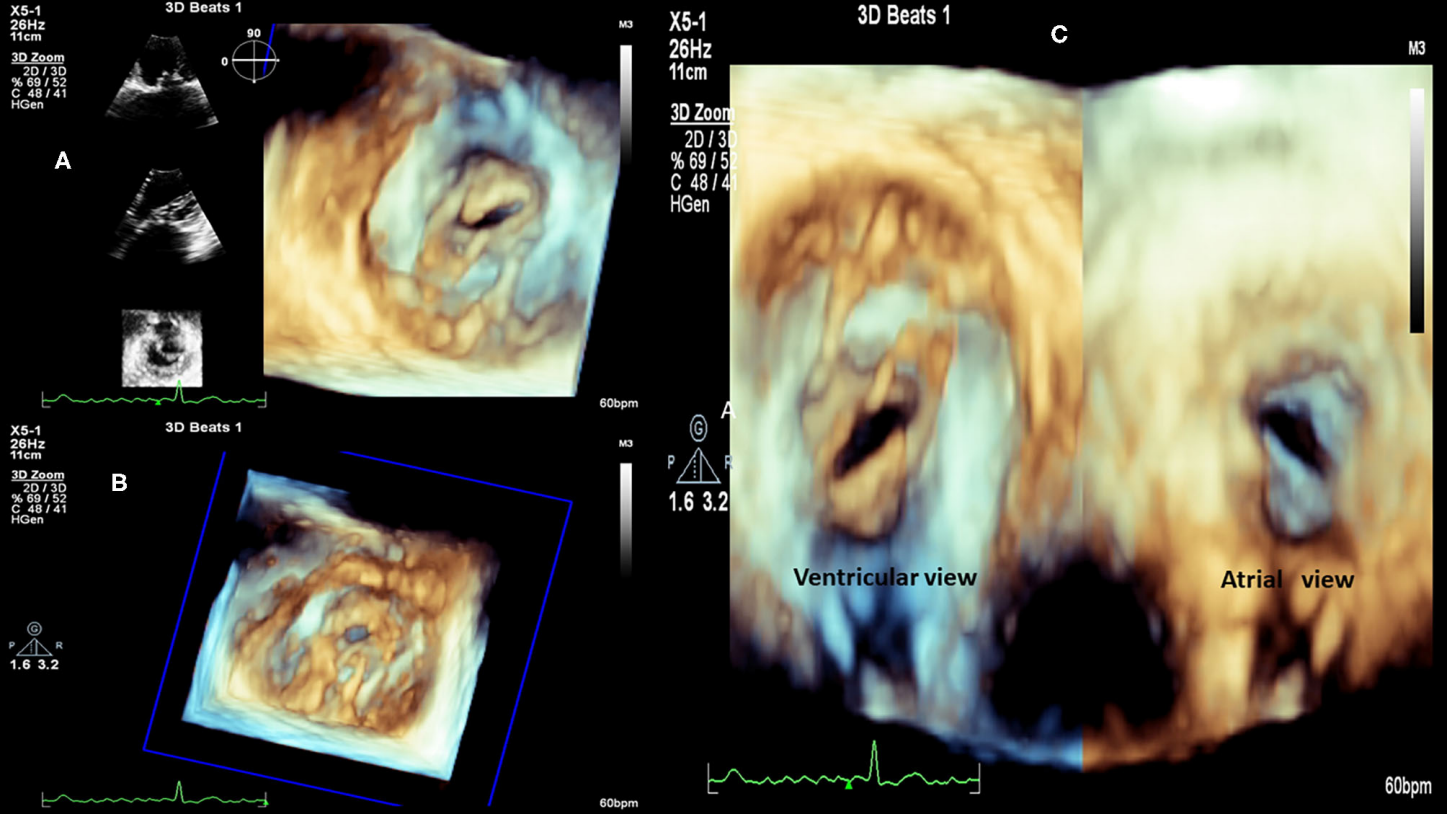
RHD (Mitral valve stenosis). (A) 3D transthoracic echocardiography, left atrium view, 2D reference structures, patient with severe valve mitral stenosis. (B) 3D transthoracic echocardiography, patient with severe valve mitral stenosis. (C) 3D transthoracic echocardiography, view from left atrium (right) and from the left ventricle (left), patient with severe valve mitral stenosis.
